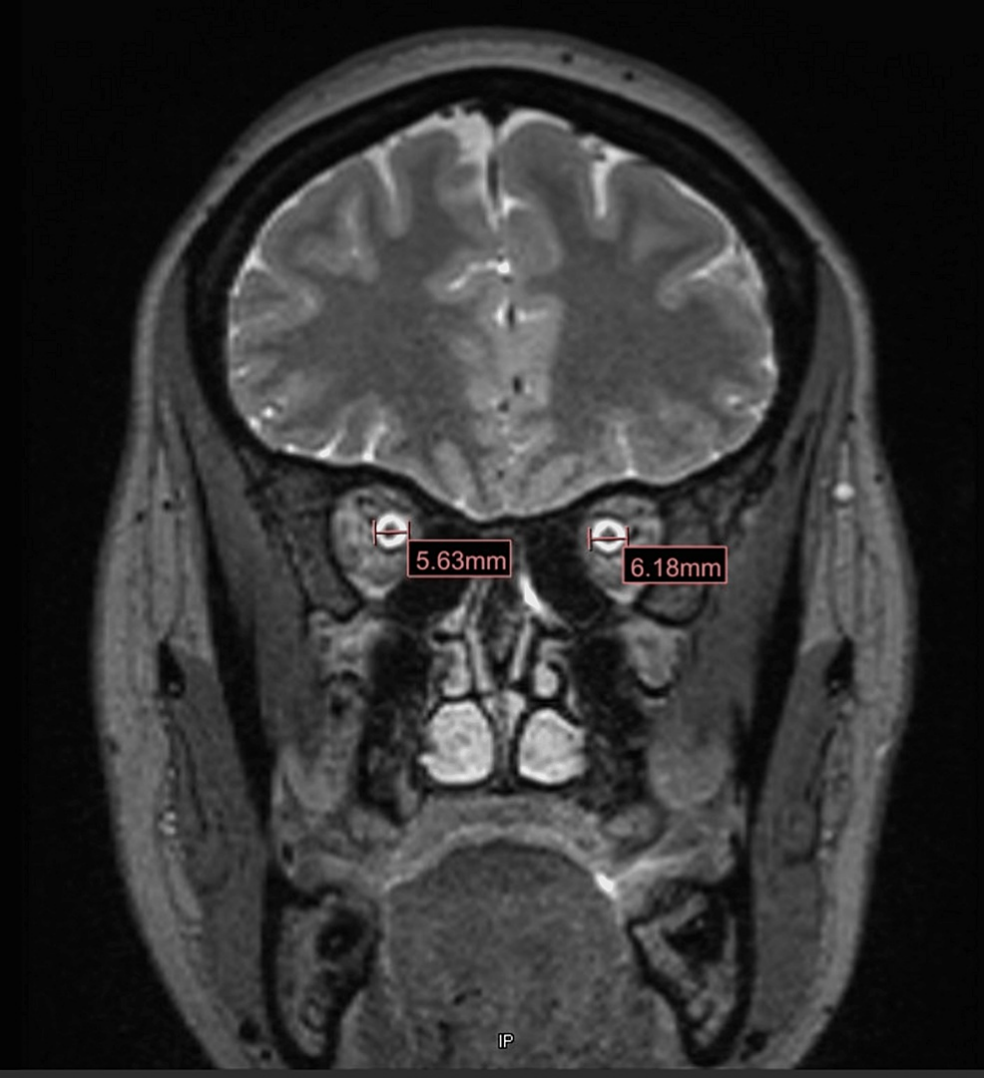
The repeat head CT scan reveals minimal mucosal lining thickening at the left side of the sphenoid sinus, bilateral middle concha bullosa, and patent ostiomeatal complexes.
